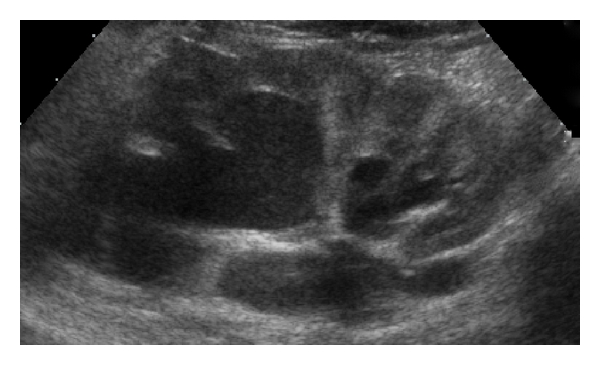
Left renal sonogram demonstrates a duplicated collecting system. Note the dilation of the upper pole moiety.
